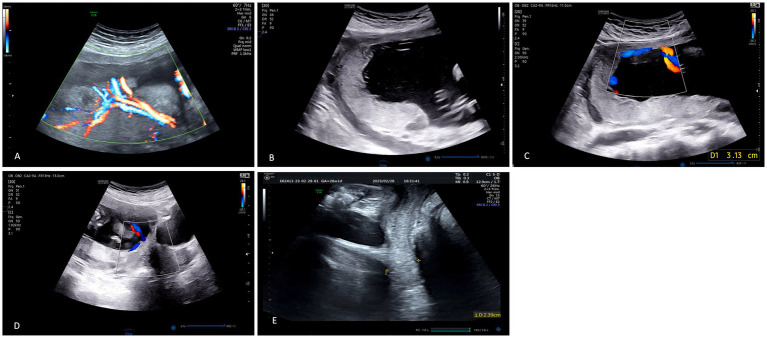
Representative ultrasound images of VCI diagnosis. (A) Color Doppler (GE Voluson E10, 23 + 0 weeks) showing fan-like vessel distribution. (B) Gray-scale (Samsung HERA W10, 22 + 2 weeks) demonstrating cord insertion >2 cm from placental margin. (C) Measurement of intramembranous length (31.3 mm). (D) Vasa previa detection (17 + 3 weeks). (E) Cervical length measurement (23.9 mm).
